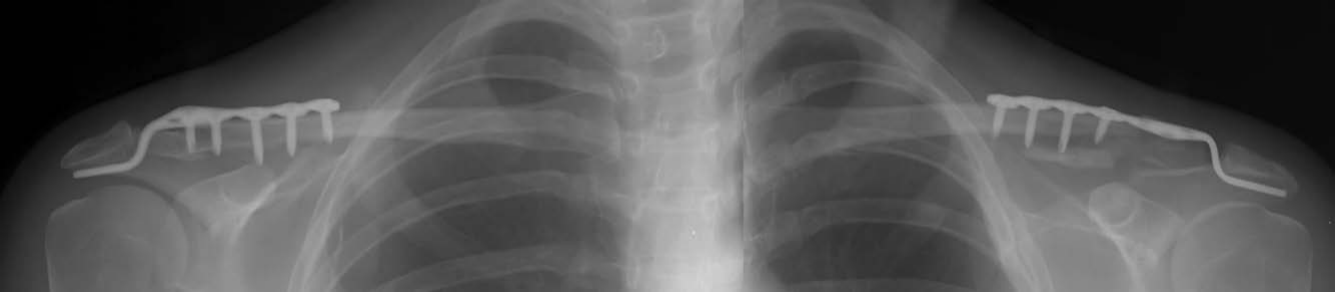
Postoperative early-period radiography (first month).
